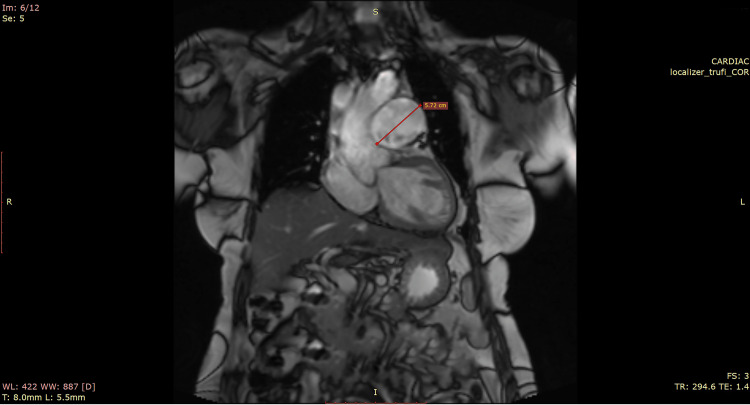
Preoperative cardiac magnetic resonance imaging from November 2021 The image shows a pulmonary artery aneurysm of 5.8 cm.
